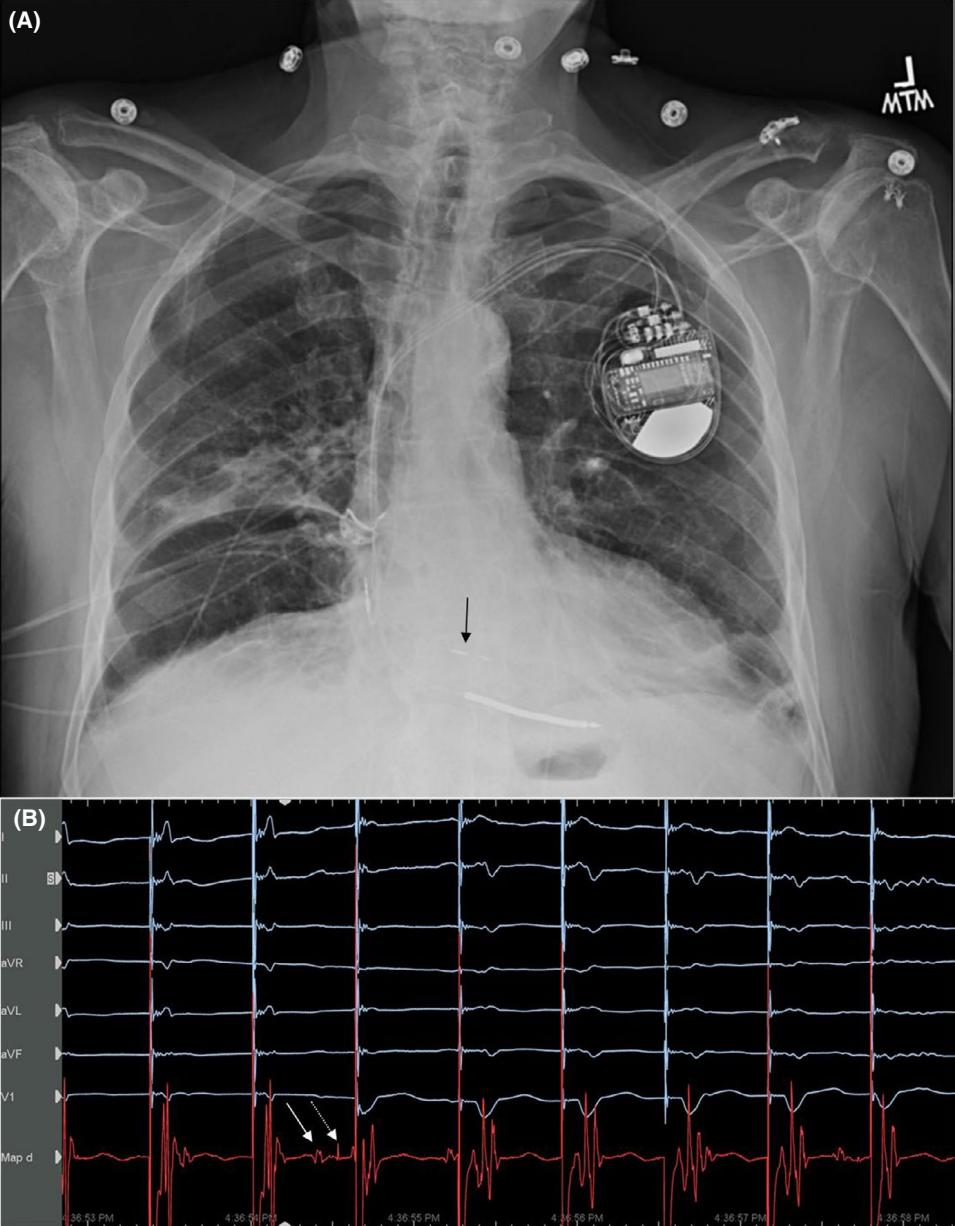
A, PA chest radiograph demonstrating pacing lead in the His‐bundle region (black arrow). B, Intracardiac lead recording demonstrating His‐bundle pacing at location depicted in panel A. The first four beats have direct His capture with a short isoelectric baseline and narrow QRS. The 5th beat demonstrates fusion. An atrial electrogram (solid arrow) followed by His‐bundle electrogram (dashed arrow) is seen. The QRS has a fused morphology between His‐bundle capture and loss of His‐bundle capture. With continued pacing, loss of His‐bundle capture with myocardial capture alone is noted. This was a repeated occurrence
